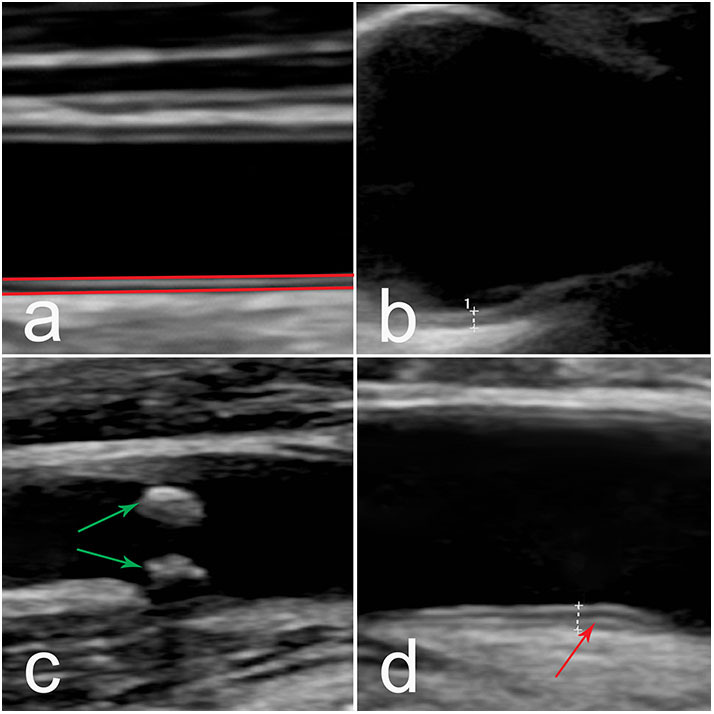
(a) Ultrasound image of the CCA. Principle of IMT measurements (red lines) in the vessel. (b–d) Ultrasound images of different vessel wall appearance, (b) smooth medium echogenic, homogenous wall thickening in the aortic arch, (c) atherosclerotic plaque (green arrow) in CCA bifurcation, (d) medium echogenic, homogenous wall thickening with fibrotic stripes (red arrow) in CFA. IMT, intima-media thickness; CCA, common carotid artery; CFA, common femoral artery.
